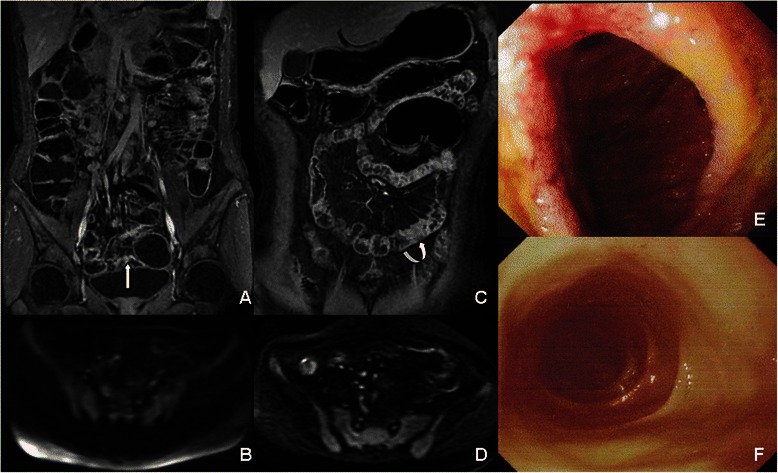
The combination of MRE and DWI could improve diagnostic sensitivity and specificity. A 34-year-old female patient suffered from active CD with multi-segmental lesions in ileum (A, B and E). On LAVA image (A) abnormal enhancement could be detected in pelvis (white arrow), which was confirmed by enteroscopy (E), While on DWI (B), no abnormal high signal intensity lesion was found in the same cross-sectional slice. A 42-year-old male patient suffered from active CD (C, D and F). On LAVA image, suspicious local bowel wall thickening (curved arrow-head) was detected in distal ileum (C), while on DWI (D), no high signal intensity was found in the same cross-sectional slice, which was confirmed by enteroscopy (F).
